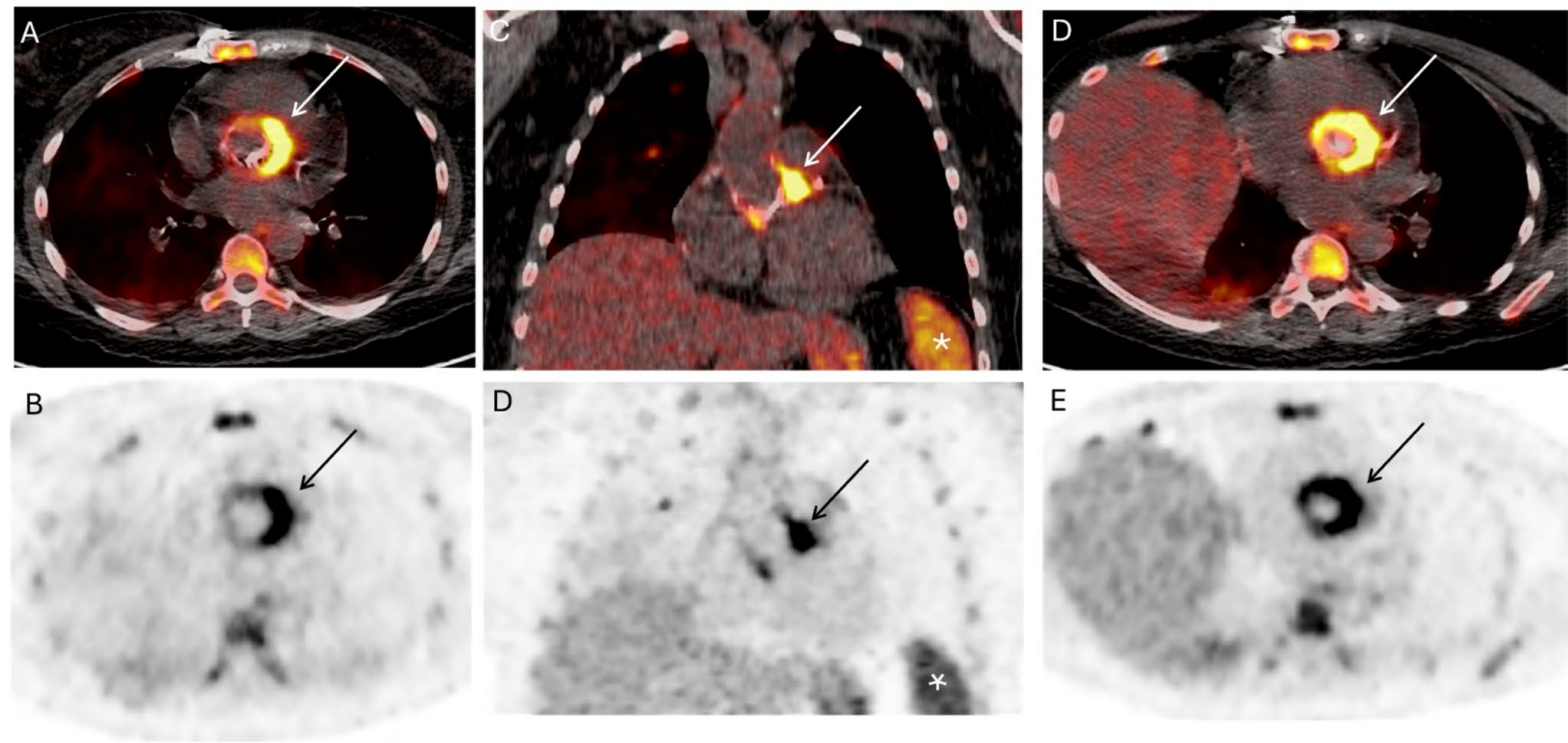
Aortic prosthetic valve infective endocarditis. A 52-year-old female presented with fever and bacteremia in the context of a mechanical aortic valve. A ^18^F-fluorodeoxyglucose (^18^F-FDG) PET/CT imaging study was acquired, obtaining images from the vertex of the skull through the toes, followed by a dedicated cardiac bed acquisition. Fusion and PET images are displayed, images A and B correspond to the axial plane, images C and D correspond to the coronal plane, and images D and E are images oriented to the aortic valve plane. There is intense semi-concentric uptake along the ring of the mechanic aortic valve, higher along the left portion of the valve, concerning for mechanical aortic valve infective endocarditis (*white arrows* on fusion images and *black arrows* on PET images). Additionally, there is increased bone marrow and splenic uptake, most likely related to the underlying inflammatory/infectious systemic process (*white asterisks* on images C and D)
